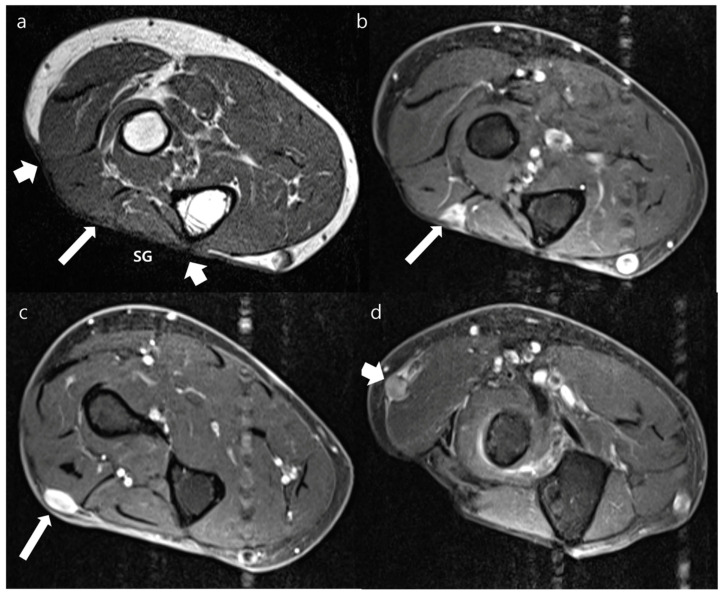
Postoperative MRI obtained from the forearm of an 88-year-old woman who underwent surgical resection of myxoid liposarcoma with immediate skin graft at the forearm 4 years prior. (a) The axial T1WI shows skin graft (SG) with peripheral (short arrows) margins on the dorsal side of forearm. An isointense nodule involves the deep margin of the transplanted tissue (arrow), which was not well delineated because of similar signal intensity with that of perilesional muscle. (b) Axial contrast-enhanced FS-T1WI obtained at the same section with (a) shows moderate enhancement of lesion (arrow) at the deep margin of SG. (c) Axial contrast-enhanced FS-T1WI obtained 2.4 cm distal to (a,b) shows another lesion arising from peripheral margin (arrow). This lesion has a well-defined nodular appearance with intense enhancement. (d) Axial contrast-enhanced FS-T1WI obtained 2.0 cm proximal to (a,b) shows another lesion in the regional location, which was apart from transplanted tissue (arrow). This lesion has a well-defined nodular appearance with moderate enhancement. These three lesions were confirmed as recurrence of myxoid liposarcoma via excisional biopsy.
